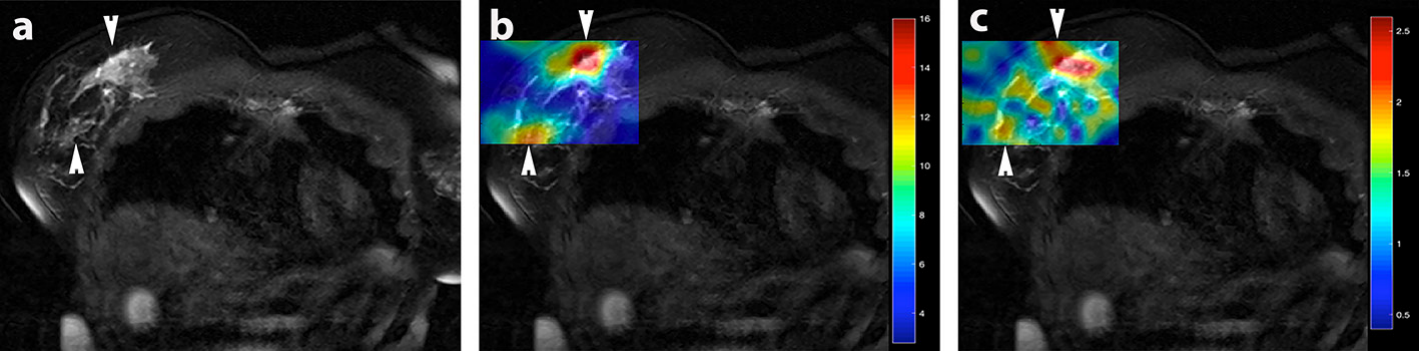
HP-MRI: Coronal *T_2_* weighted MRI with rectangular ^13^C metabolite colour map superimposed. Red denotes regions of highest metabolite concentration whereas blue denotes the lowest. (a) *T_2_* weighted coronal image of the tumour. The regions of tumour are highlighted with white arrowheads. The respective metabolic maps are, (b); pyruvate map, and (c); lactate map. Images are from ^13^C CSI first acquisition.
